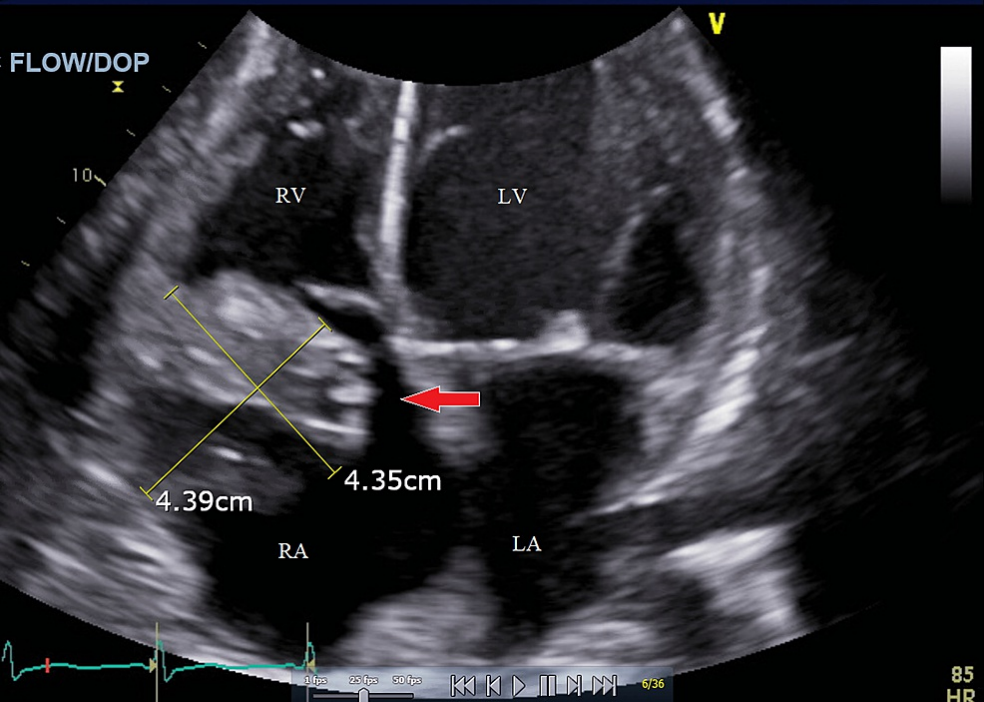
Transthoracic echocardiogram via four-chamber view with a red arrow pointing to the vegetation before the AngioVac procedure. RA, right atrium; RV, right ventricle; LA, left atrium; LV, left ventricle
